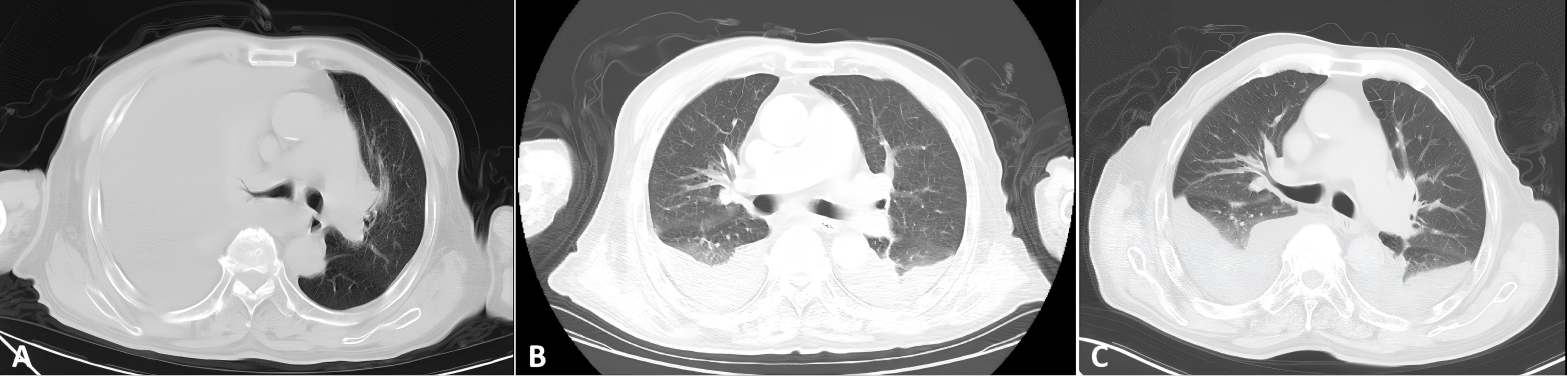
Serial bilateral chest CT imaging of pleural effusion. (A) February 2025: Image obtained at initial discovery. (B) March 2025: Pre-treatment follow-up scan. (C) April 2025: Scan obtained after one cycle of chemotherapy.
